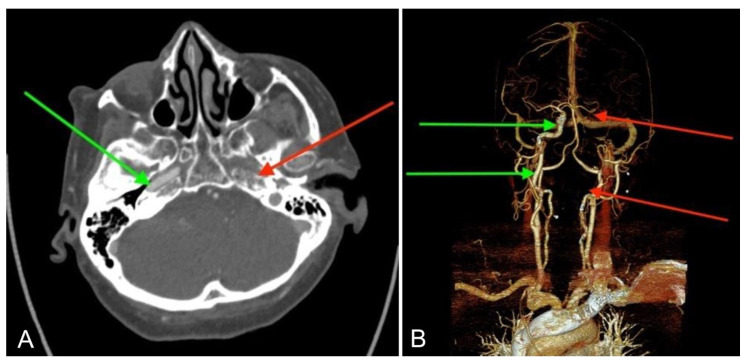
Traumatic occlusion of the left ICA of case 4. CT with contrast 24 h after the middle ear surgery, 1 h after the onset of stroke symptoms. Visible normal, patent right ICA (green arrows) and completely occluded left ICA (red arrows). Axial scan (A) and reconstruction of the head and neck arteries (B).
